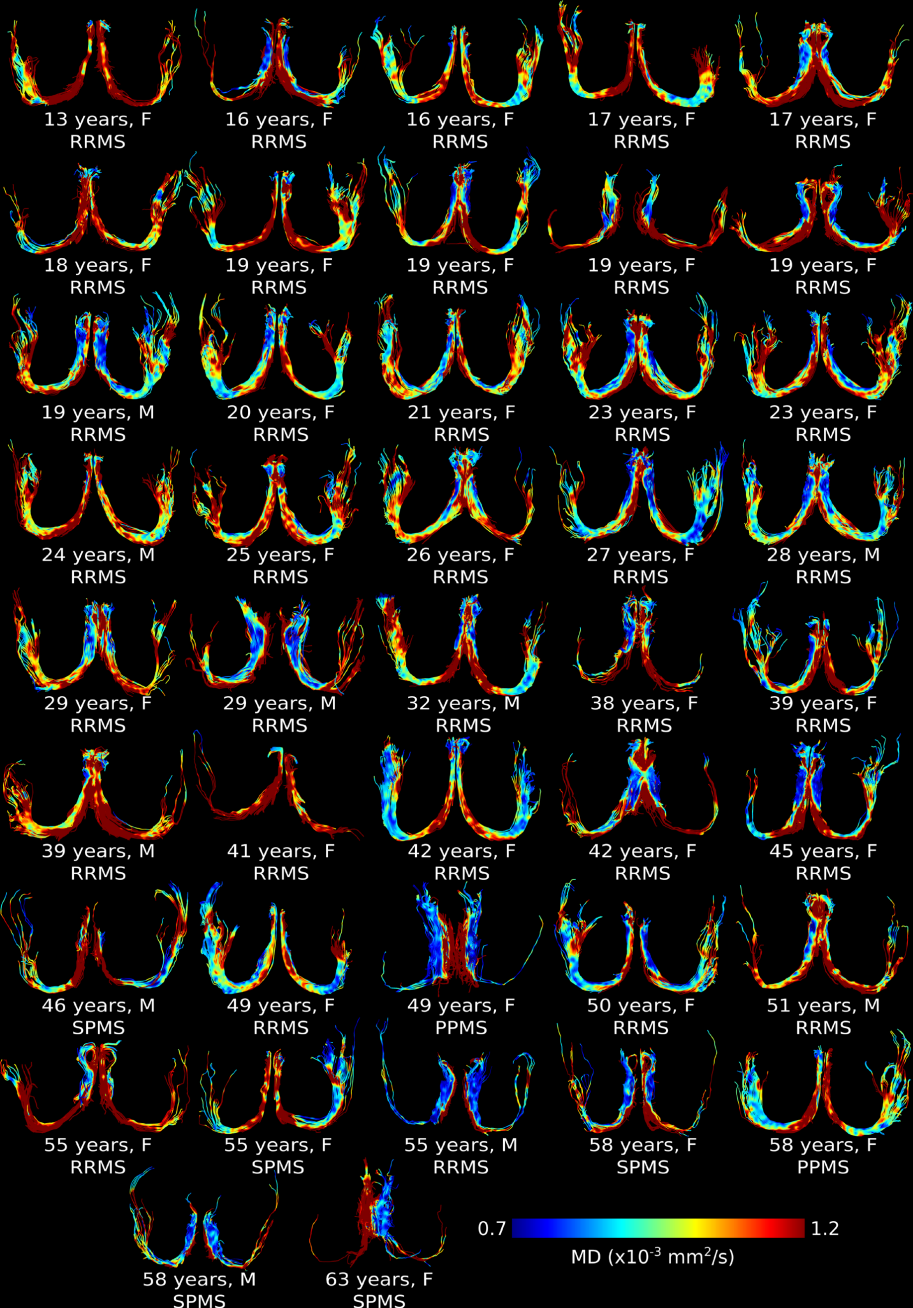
Example of CSF-suppressed, high-resolution FLAIR-DTI fornix tractography (as seen from superior view) color-coded by MD in all 42 MS, ordered by age. Fornix tractography depicted the full fornix in all, but it appeared thinner and showed bilateral regions with higher MD at most ages.
